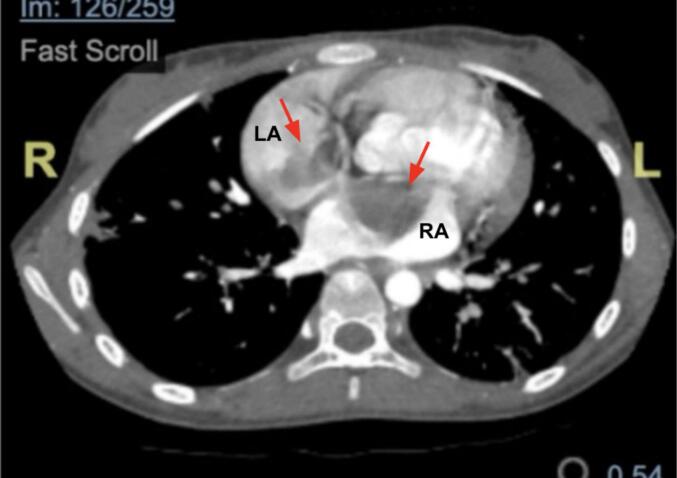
Cardiac CT showing dilated RA and LA with hypodense lesions (red arrows) measuring approximately 3,67 × 4,0 cm in the RA and 2.49 × 3.73 cm in the LA. LA (left atrium); RA (right Atrium).
